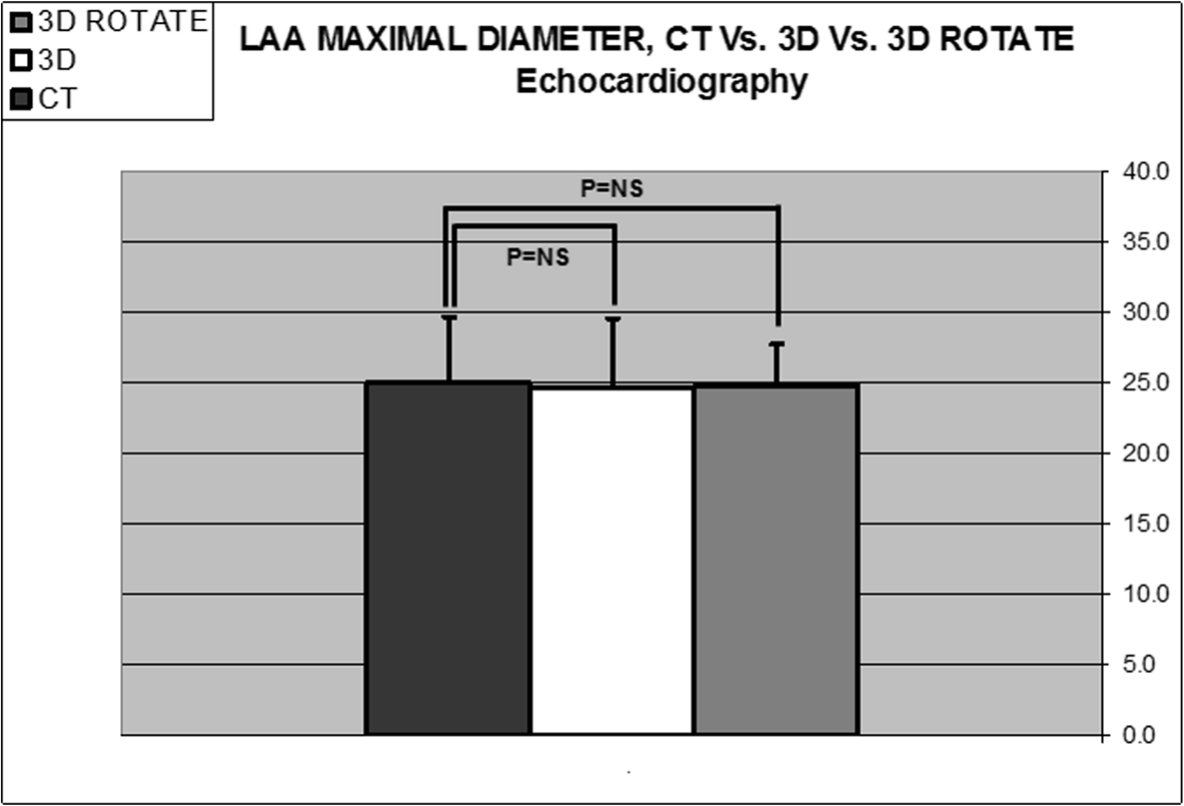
Histogram comparing LAA maximal diameter using different imaging methods (*n* = 41). Comparison between computed tomography (CT) (24.9 ± 5.8 mm), direct Real-Time 3-Dimensional Transesophageal Echocardiography (3D) (24.6 ± 5.0 mm), and Rotational 3DTEE (3D Rotate) (24.8 ± 4.5 mm), (*p* = NS for all)
